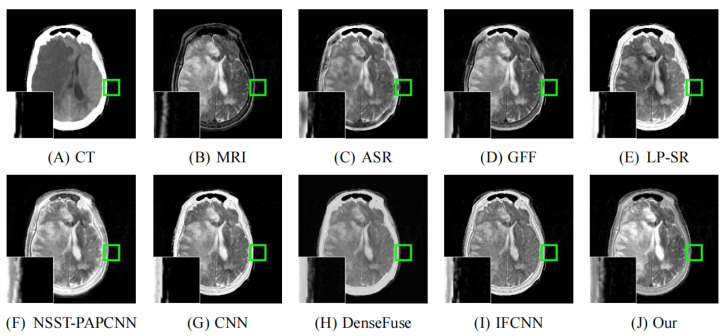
Fusion images of “Fatal stroke-14” by various methods. From (A) to (J): CT, MRI, ASR, GFF, LP-SR, NSST-PAPCNN, CNN, DenseFuse, IFCNN, Our proposed method.
